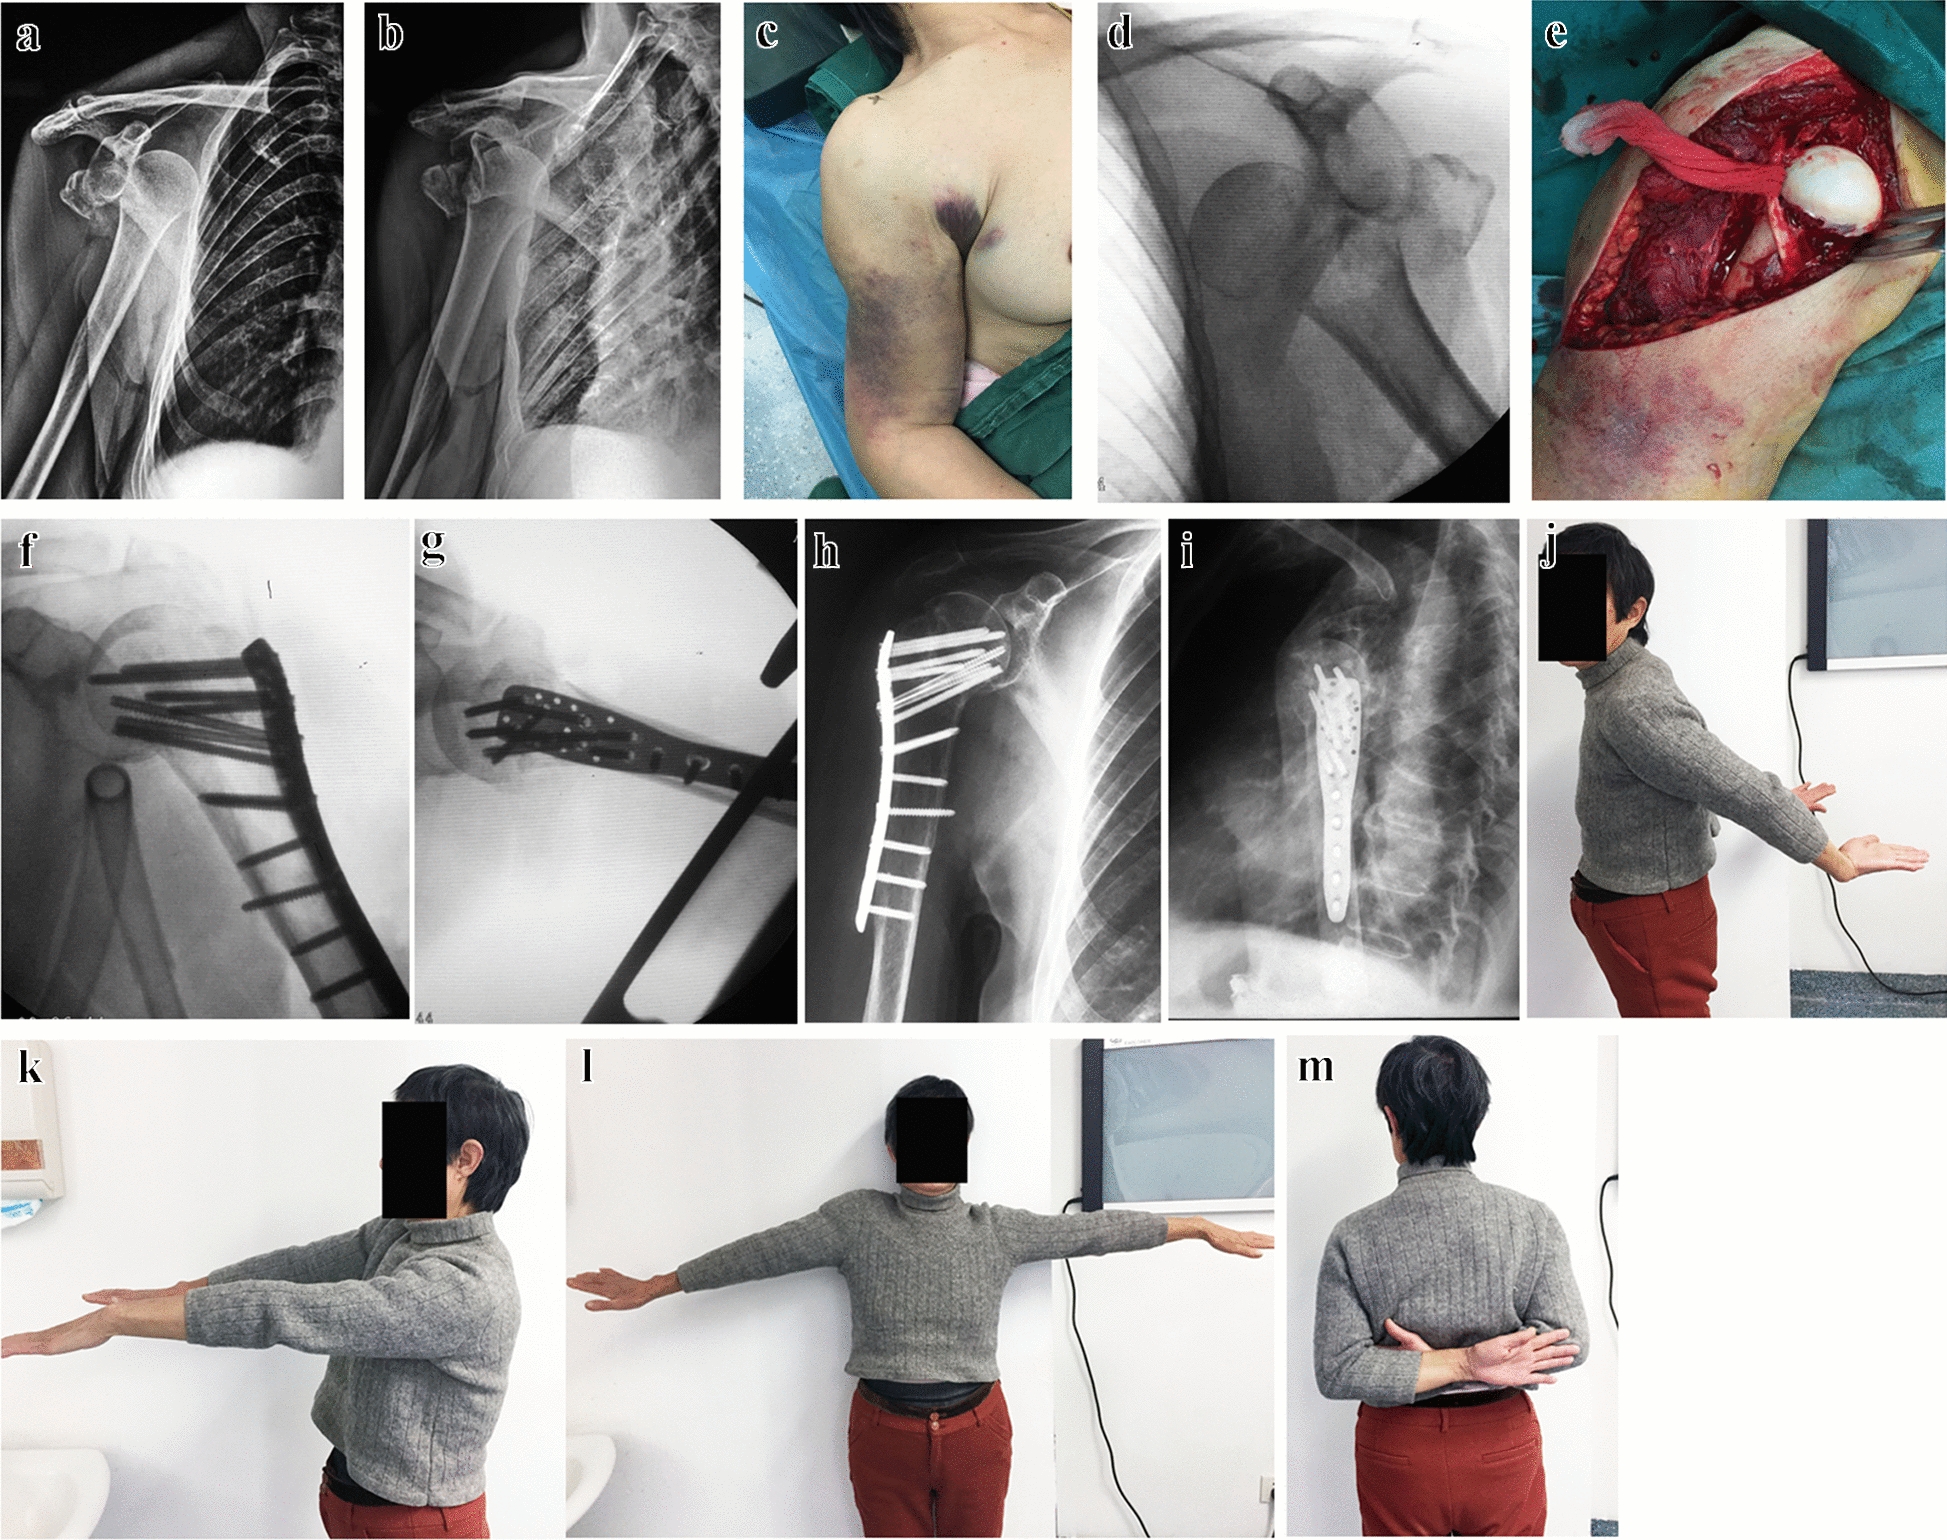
Iatrogenic fracture occurred and revision surgery. a, b Preoperative radiographs. c General appearance. d Iatrogenic fracture occurred. e Intra-operative situation. f, g C-arm fluoroscopy. h, i Postoperative radiograph at 12 months. j–m Range of motion at 12-month follow-up
